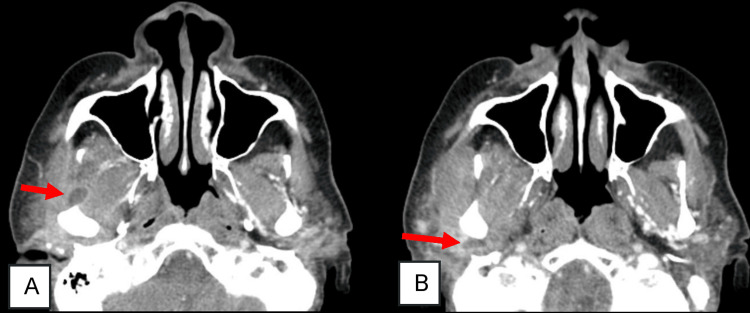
Computed tomography (CT) scan before treatment CT scan revealing two small abscess collections (red arrows) surrounding the right mandibular condyle, anteriorly (A) and posteriorly (B)
